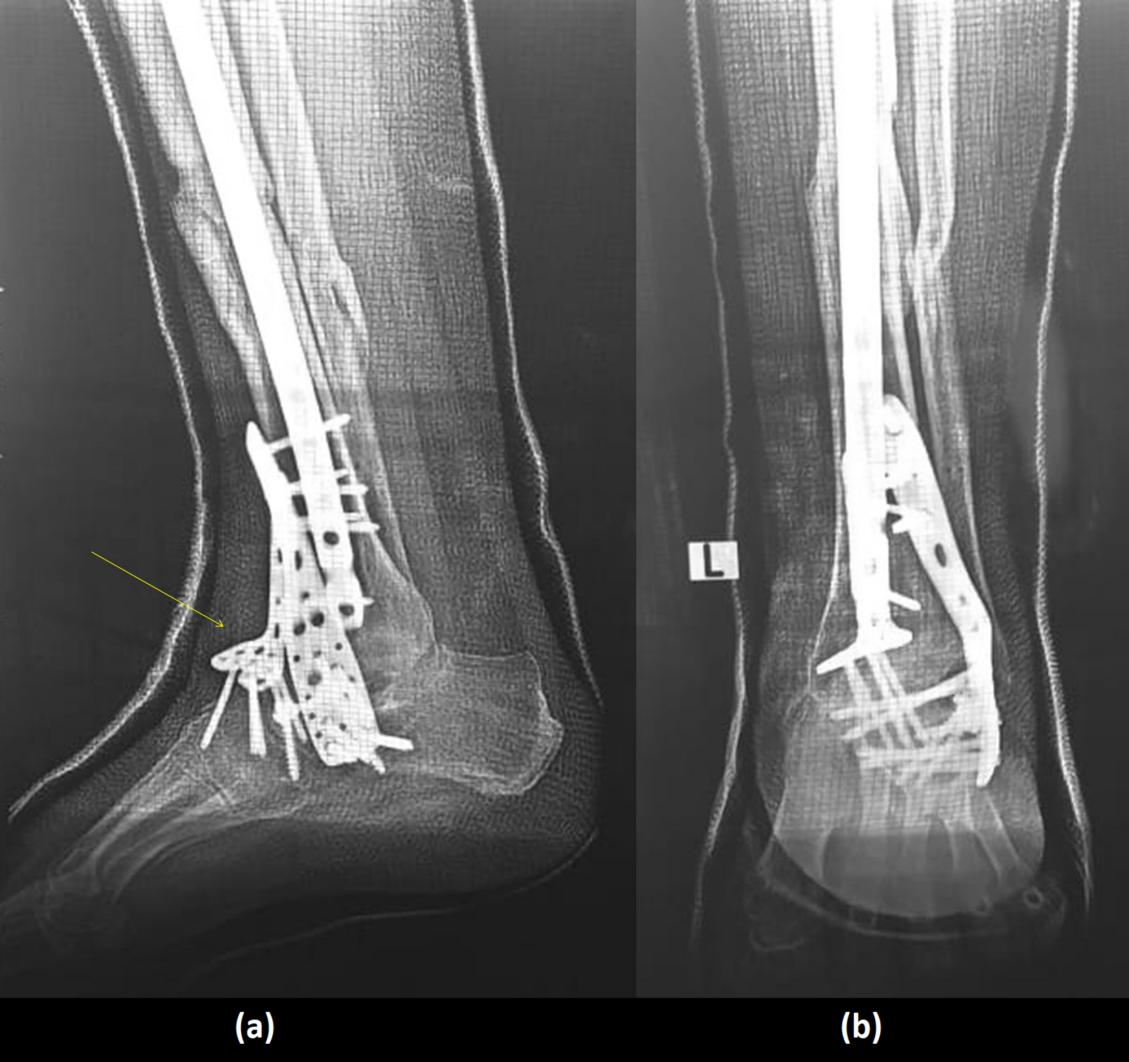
Radiographs post-tibiocalcaneal arthrodesis: (a) anteroposterior view and (b) lateral view.
